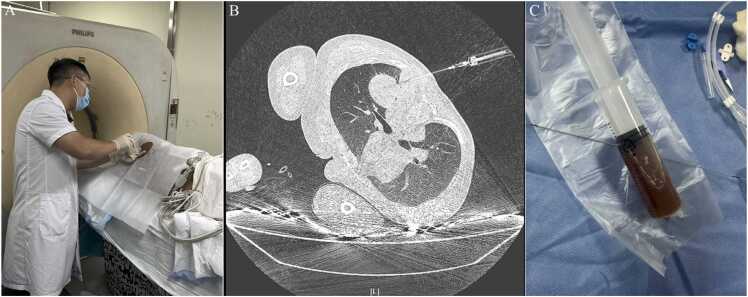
Percutaneous Drainage of the Pulmonary Abscess Under CT Guidance. (A) The procedure was performed in the CT suite, beginning with the administration of local anesthesia. (B) An axial CT image confirms the precise placement of the needle tip within the thick-walled abscess cavity, with clear avoidance of adjacent critical structures. (C) Aspiration of the cavity yielded thick, dark brown purulent fluid.
